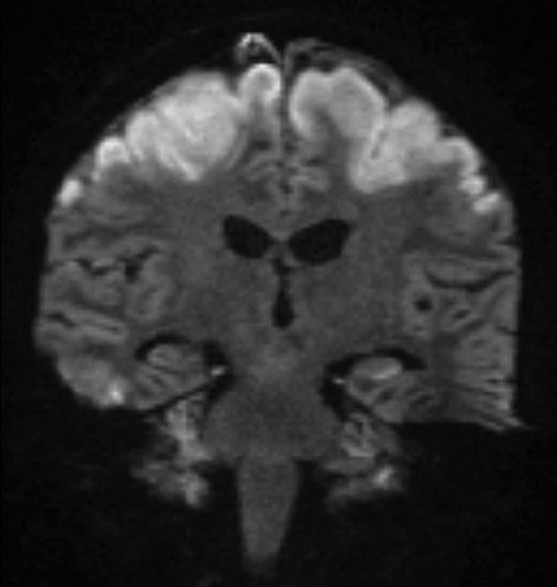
Diffusion weighted MRI, day 5 in ICU
